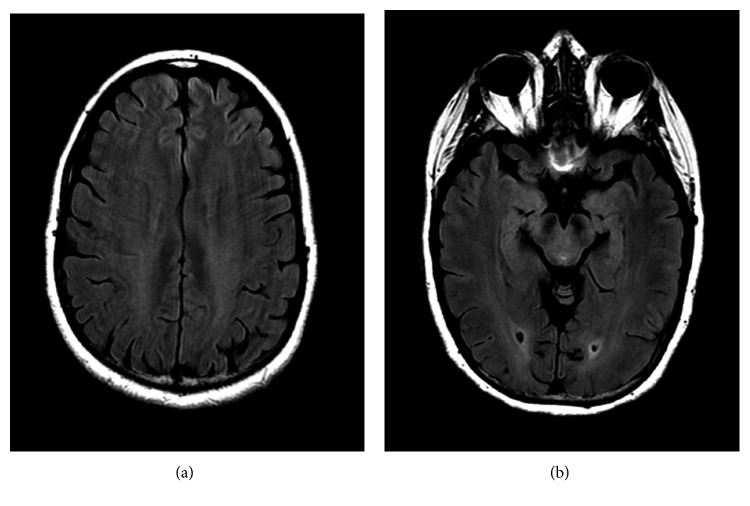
Axial FLAIR images of the brain demonstrating complete resolution of previously noted hyperintense signals in the (a) posterior right parietal lobe and (b) posterior occipital lobes bilaterally.
